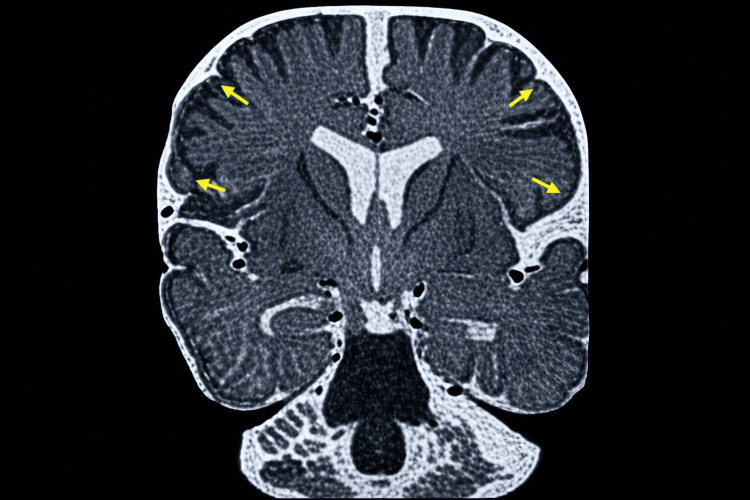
Coronal brain magnetic resonance imaging The MRI image shows fronto-temporo-parietal cortico-subcortical atrophy with widened cortical sulci and enlarged extra-axial cerebrospinal fluid spaces (yellow arrows), consistent with diffuse cerebral atrophy.
